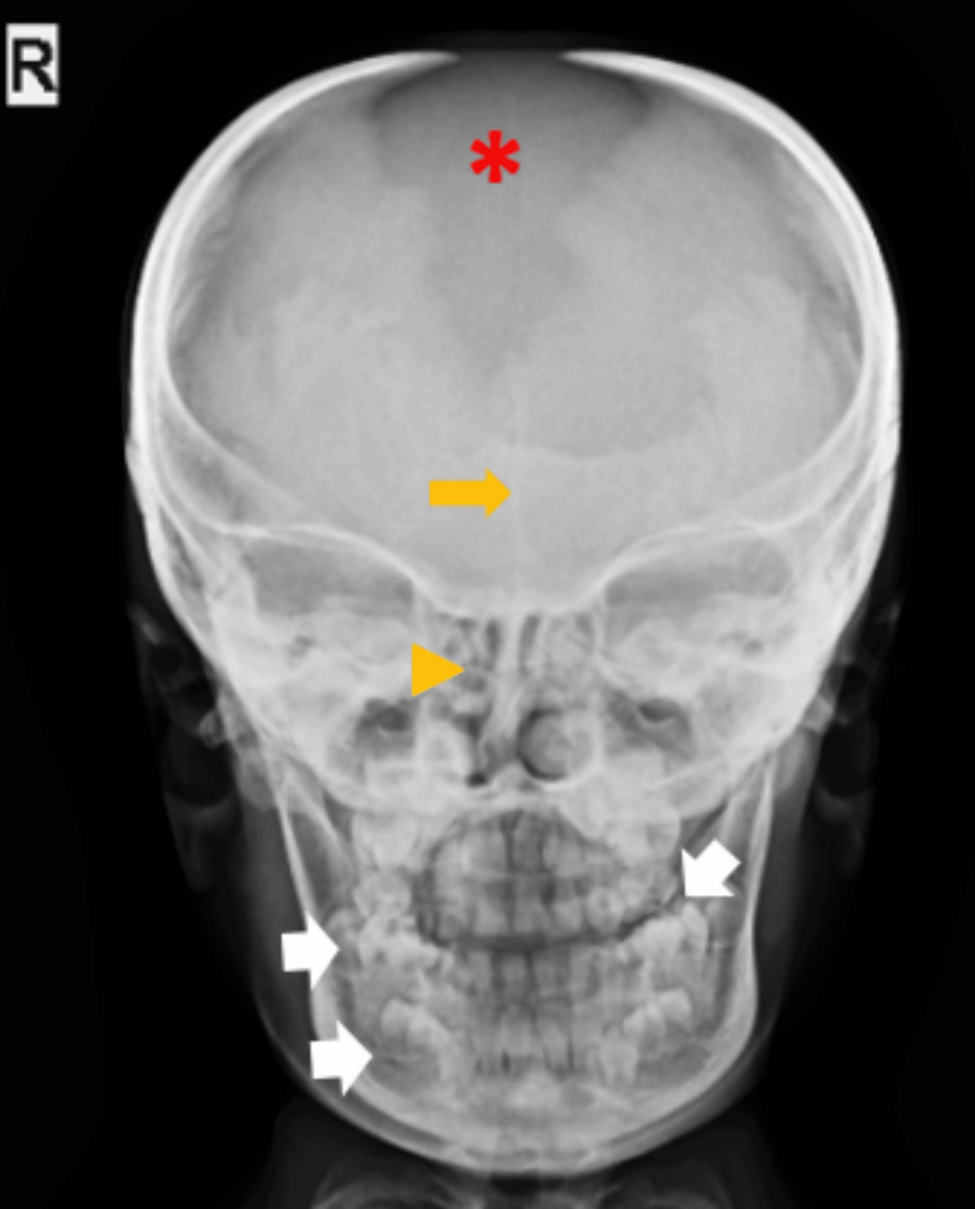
Frontal skull radiograph demonstrating a persistent metopic suture (arrow), absent nasal bones (arrowhead), a wide-open anterior fontanelle (red asterisk), and multiple supernumerary teeth (white arrows).
